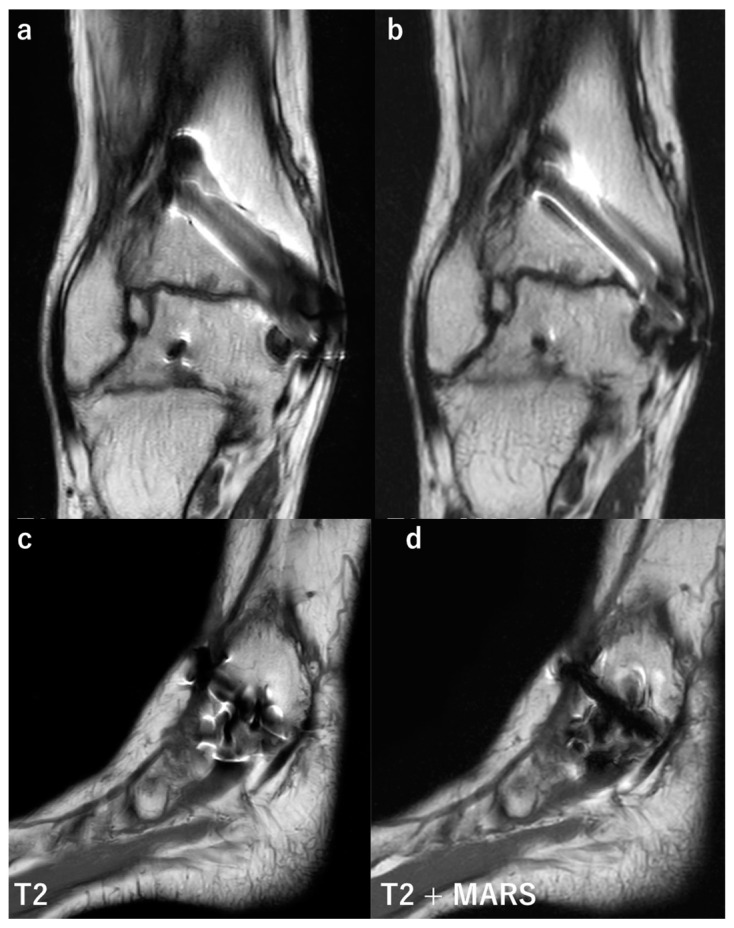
Comparison of conventional MRI and images obtained with MARS. Coronal and sagittal MR images of a patient after medial malleolus fracture of the right ankle. The fracture was treated by a screw fixation. The coronal MR image (a) was taken by conventional T2 weighted image (T2WI), and significant signal loss and distortion around the implant are present. Image (b) was taken by T2WI with MARS, showing a better visualization of tissue around the screw. The sagittal image (c,d) was taken by T2WI, and T2WI with MARS, respectively. Similarly, with coronal images, signal loss and distortion are reduced with MARS, leading to better visualization of tissue surrounding the implant.
